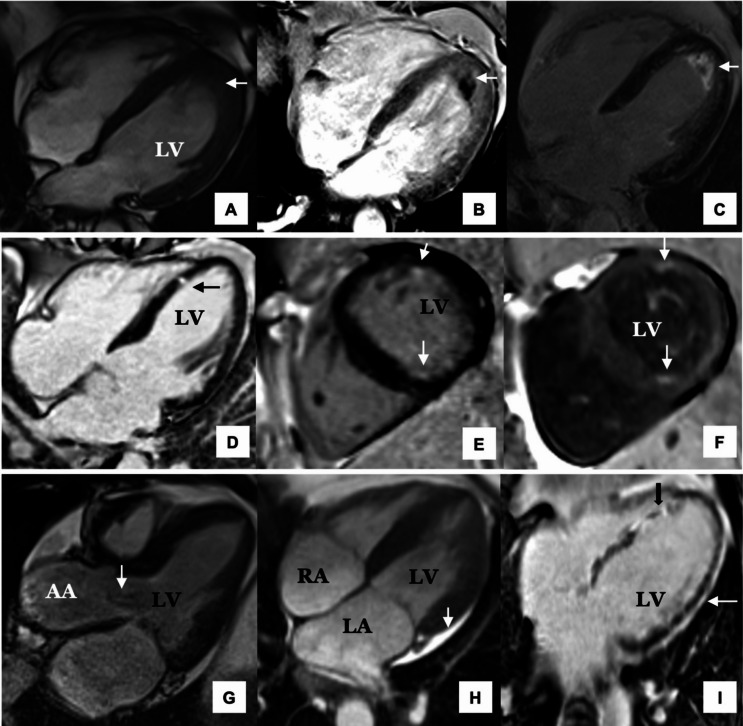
Cardiac pathology in EGPA. A-C: Thrombus– Cardiac MRI 4 chamber view showing A: thickening of the apex on the steady state free precision sequence (*arrow*). (B): In the same patient, the thrombus in the LV apex is better delineated on the early gadolinium sequence (*arrow*). (C): The same thrombus shows heterogenous LGE (*arrow*). (D-F): A series of images post gadolinium showing (D): multifocal tiny subendocardial infarcts along the septum (*arrow*). (E): Tiny subendocardial infarction inferior and anterolateral segments (*arrows*). (F): The infarcts (same patient from 3E) are better delineated on the black blood phase sensitive inversion recovery sequence (*arrows*). (G): Valvular disease– Steady state free precision sequence with the regurgitation jet across the aortic valve (*arrow*). (H): Chamber dilatation and Pericardial effusion– 4 chamber view steady state free precision sequence-left atrium (LA) is dilated. There is a small pericardial effusion (*arrow*). (I): Pericarditis– 4 chamber view shows intense subendocardial LGE along the interventricular septum (*black arrow*) and diffuse intense smooth pericardial enhancement (*white arrow*) in keeping with pericarditis. Abbreviations: LA, Left Atrium; LGE, Late gadolinium enhancement; LV, Left ventricular; MRI, magnetic resonance imaging
